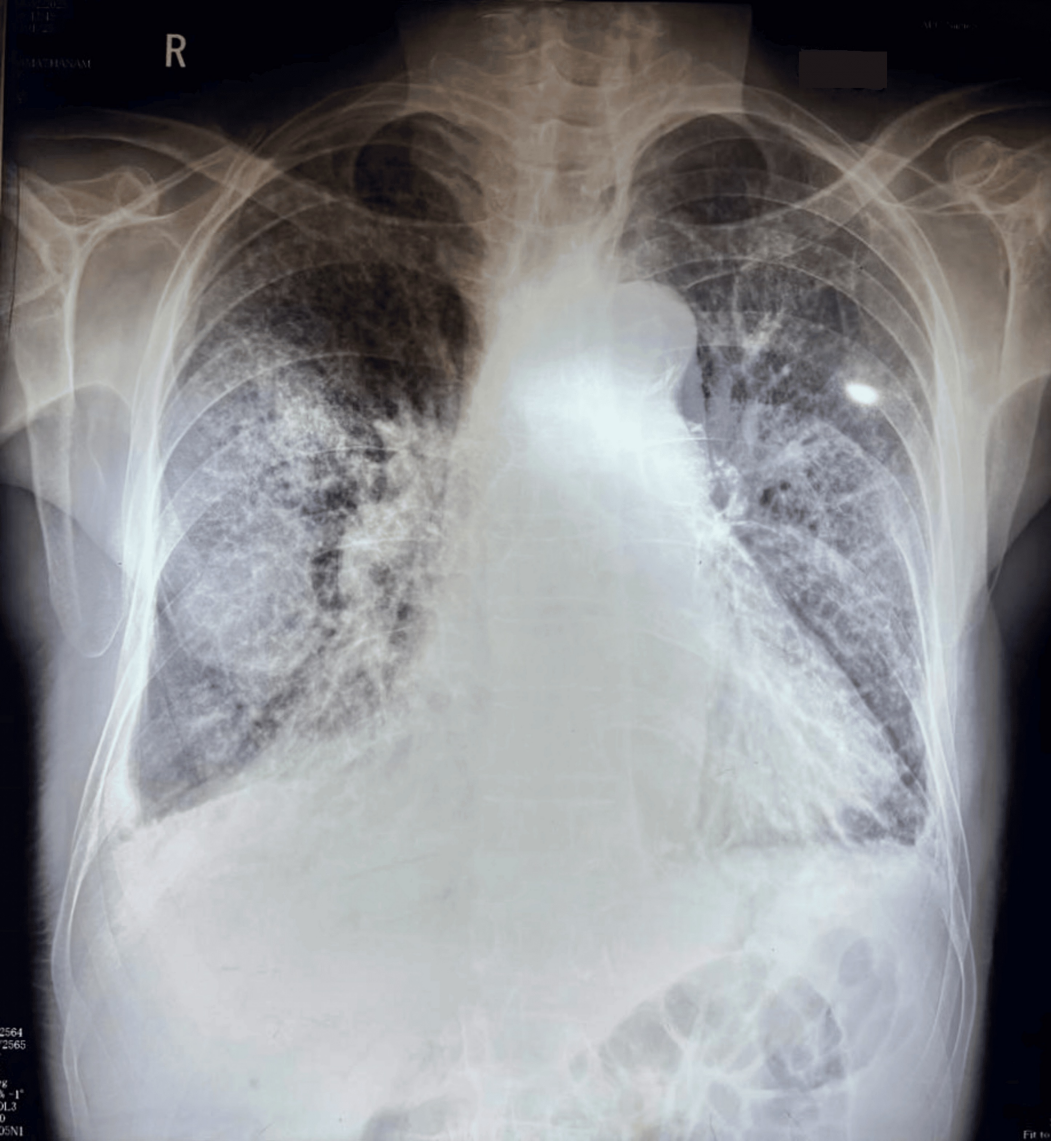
Chest X-ray shows alveolar edema with "Batwing" appearance. There were Kerley B lines and peribronchial cuffing due to interstitial edema. There were upper lobe diversion of pulmonary vessels, cardiomegaly, and bilateral blunting of costophrenic angles (pleural effusion) due to heart failure
